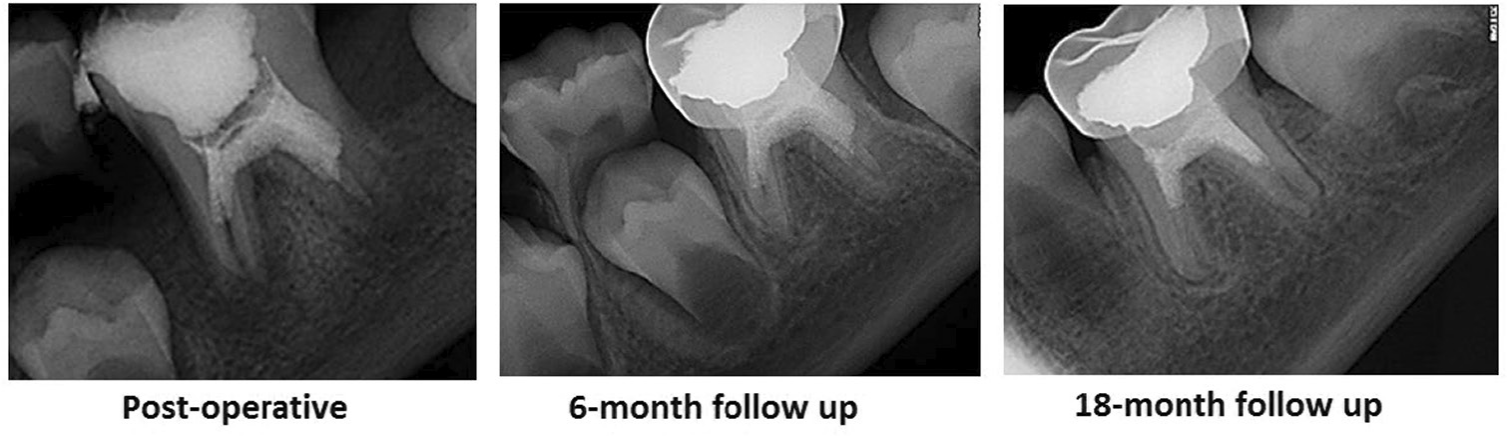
Post‐operative and follow‐up radiographs of tooth #19 after revascularization.
